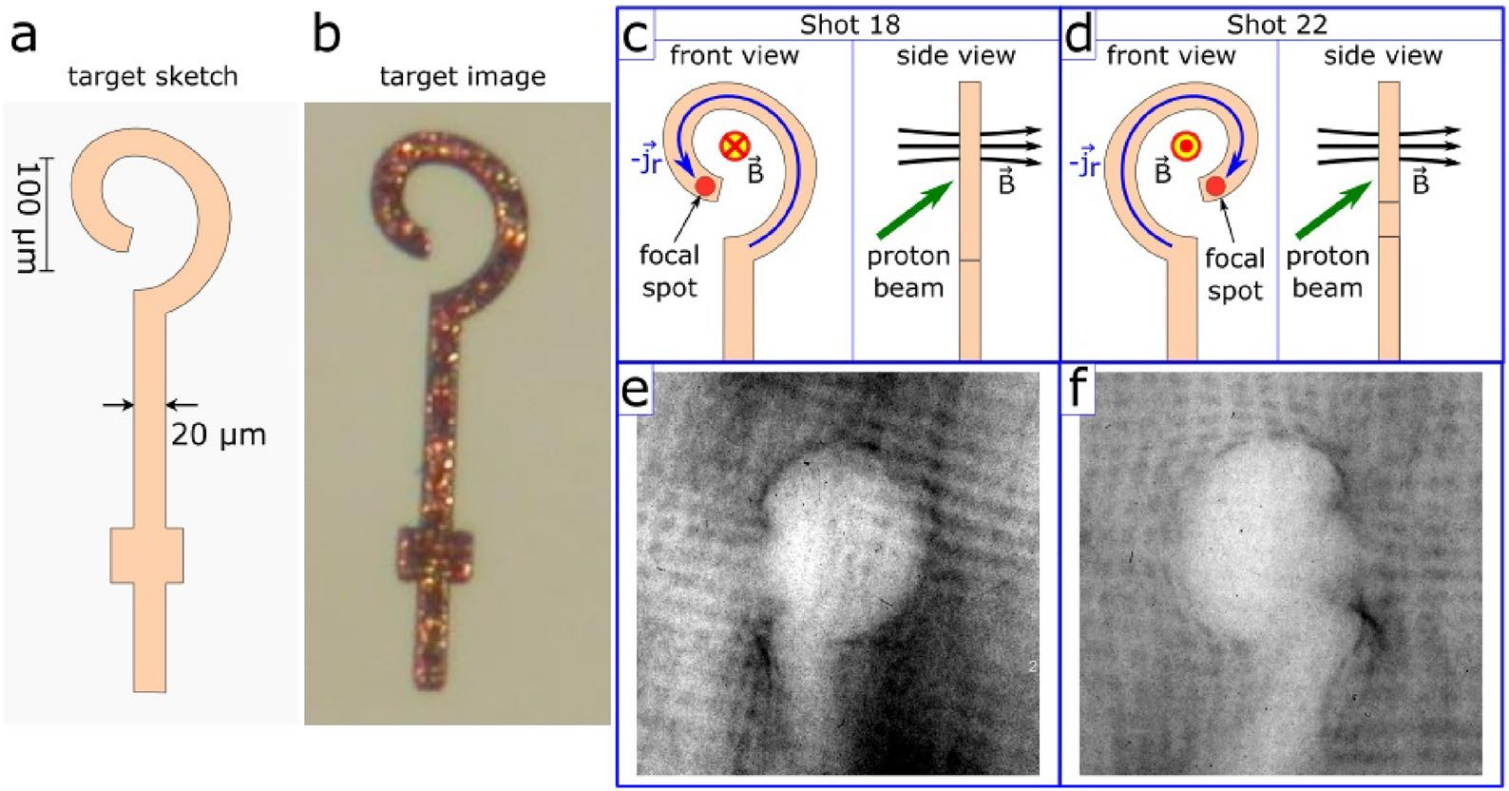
The target sketch (a) and the magnified photographic image (b). Scheme of the setup used in Shot #18 (c) and #22 (d). Proton radiography image obtained in Shot #18 in the second layer of RCF stack, corresponding to Bragg peak position for $$\approx 3$$ MeV protons, passing the studied region $$\approx 25$$ ps after the end of the laser pulse; darker colors correspond to higher proton concentrations (e). The same for Shot #22 (f).
